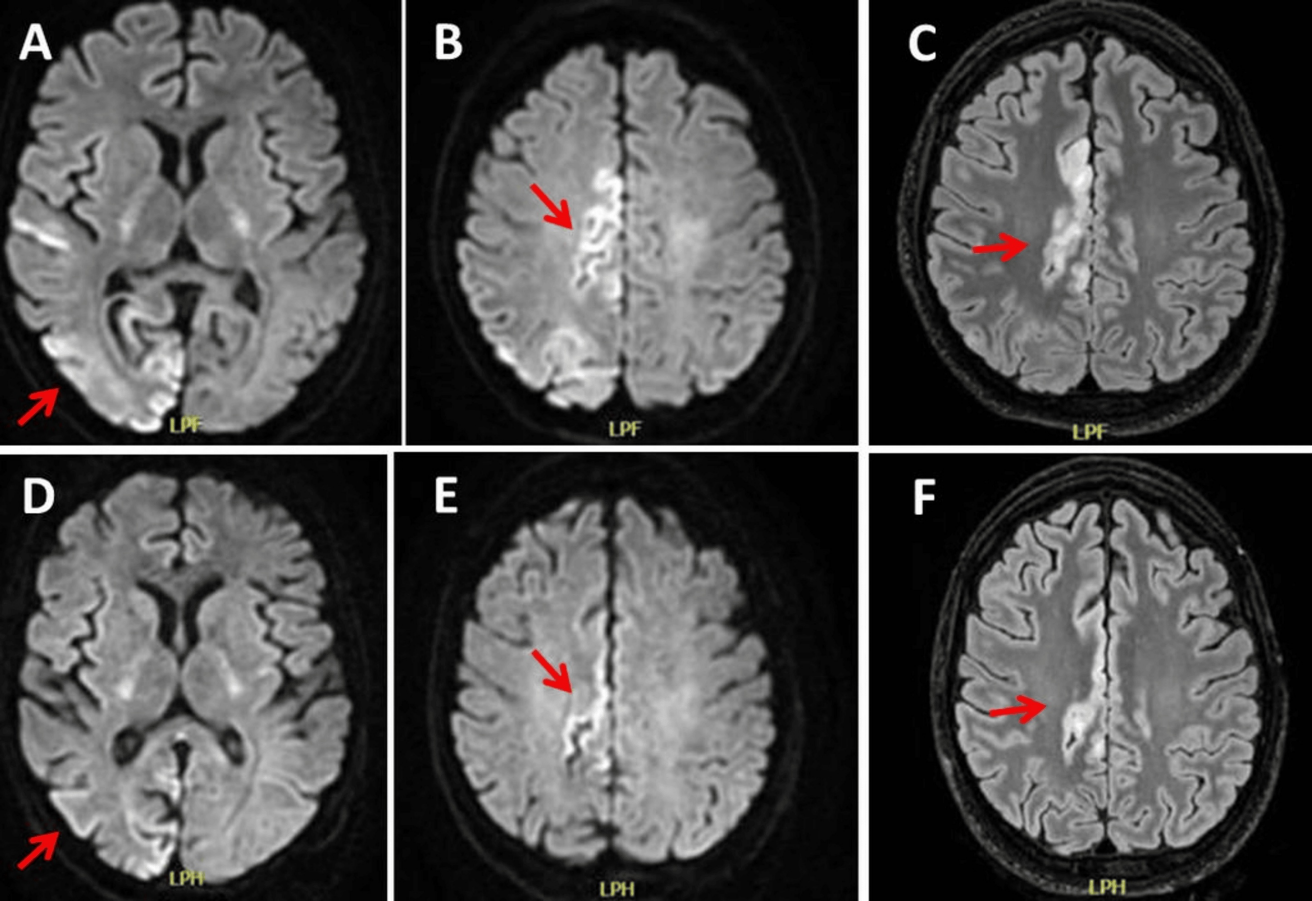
MRI of the brain on hospital day 27 (panels A-C) and on hospital day 52, showing further resolution of the SLL on T2 (panels D, E) and FLAIR (panel F) SLL: stroke-like lesion; FLAIR: fluid-attenuated inversion recovery
